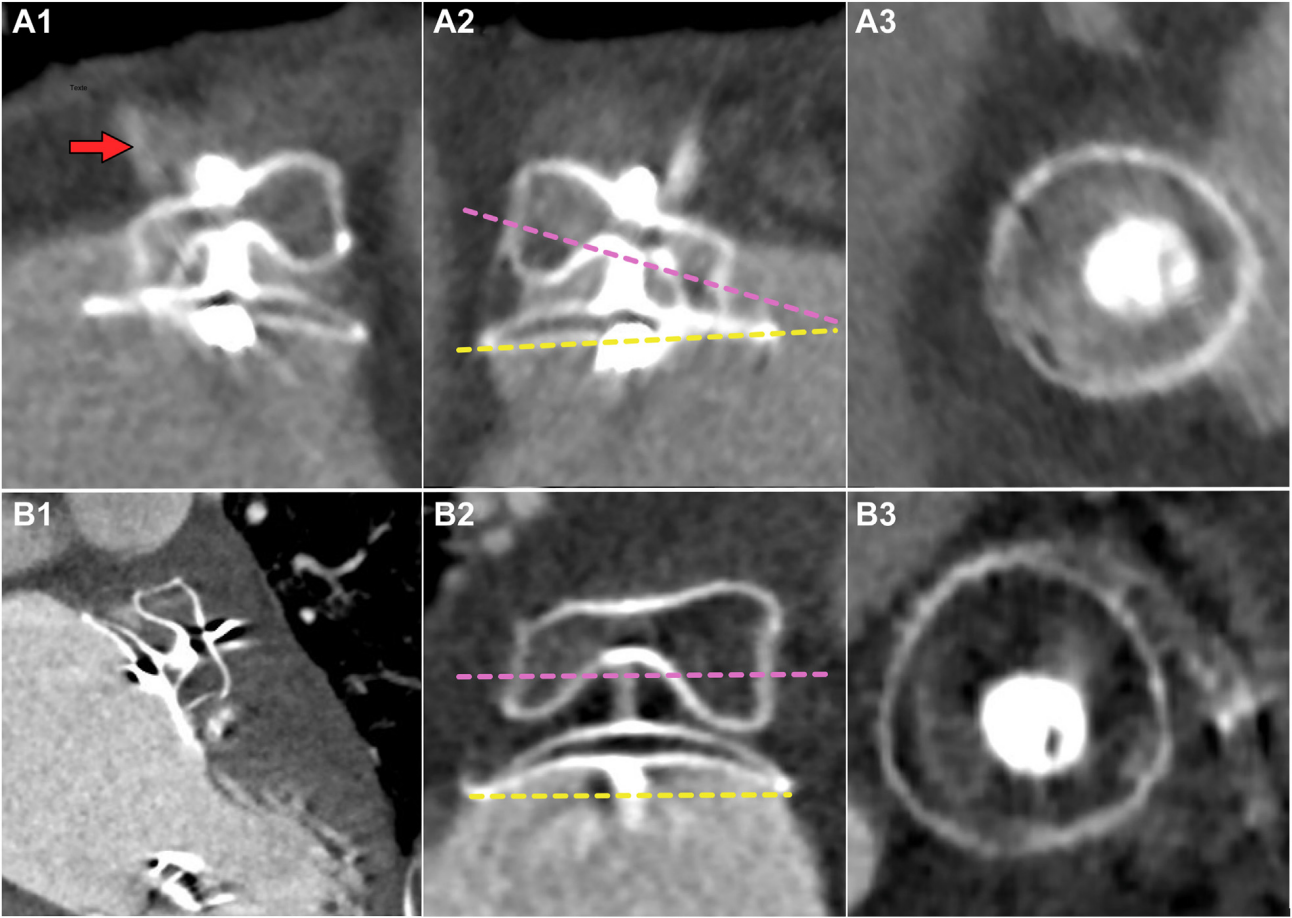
Representative examples of patients treated with the standard (A1-A3) or steerable (B1-B3) delivery sheath. Patient A had a cactus-shaped left atrial appendage (LAA), baseline index LA volume = 110 mL/m^2^, and was treated with 22 mm AMULET device implantation. The follow-up CT scan revealed an off-axis prosthesis (A1-A2) since the lobe was not perpendicular to the appendage wall on the landing zone. In addition, persistent LAA patency was observed (red arrow) in relation to a peridevice leak. Disc/lobe misalignment was also identified (A2) since the axes of the disc (dashed yellow) and the lobe (dashed purple line) intercepted with an angle >30°. Patient B had cactus-shaped LAA, baseline index LA volume = 160 mL/m^2,^ and was treated with 31 mm AMULET device implantation. The device's final position was optimal with the correct apposition of the lobe on the appendage wall. There was no residual LAA patency, nor peridevice leak observed. The disc and lobe were correctly aligned.
